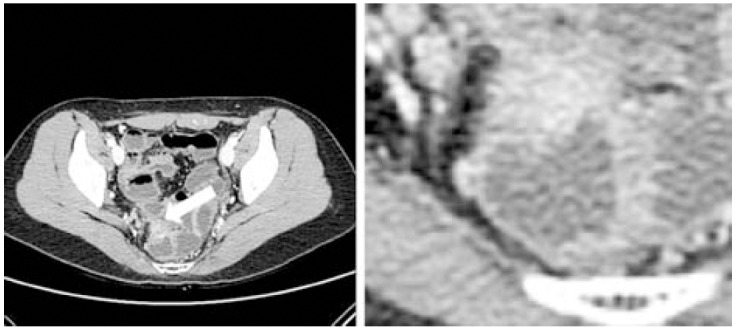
AP-CT showed a 1.5-cm sized mural nodule with heterogeneous hyperattenuation, located at mid rectum right-anterior wall; The mass was a lobulated exophytic contour with eroded mucosa & mild perirectal fat invasion. B: Magnificating view of rectal endometriosis lesion.
